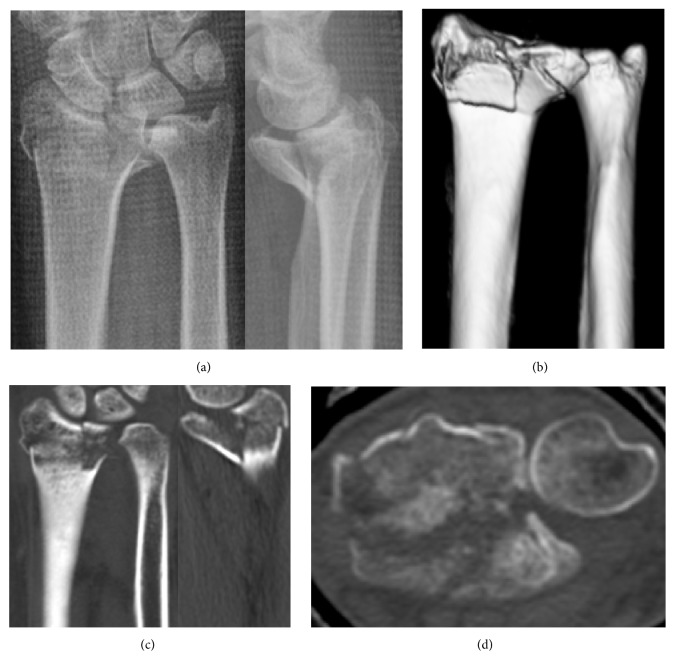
Patient 1. Plain radiographs (a) and CT (b, c, d) at the time of injury. A 10 × 8 mm VLF fragment is observed, with volar displacement.
